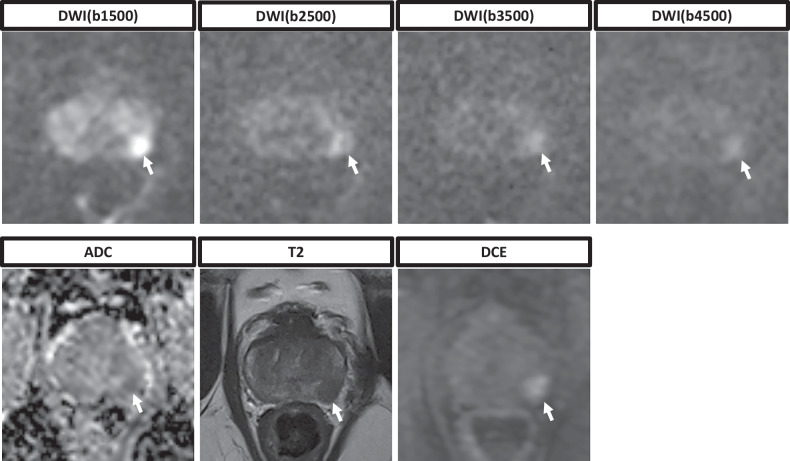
Representative images of a 61-year-old patient. The patient presented with an elevated prostate-specific antigen of 4.27 ng/mL. The whole multiparametric MRI protocol is shown, consisting of T2-weighted sequences, DWI sequences with calculation of the ADC map, and DCE sequences. The suspicious lesion in the posterolateral peripheral zone can be clearly delineated in the DWI(b1500), whereas both contrast and signal-to-noise ratio decrease in the DWI(b2500), DWI(b3500), and DWI(b4500). All readers gave a PI-RADS score of 4 for both readings that included either the DWI(b1500) or DWI(b2500). ADC, Apparent diffusion coefficient; DCE, Dynamic contrast enhanced; DWI, Diffusion-weighted imaging; MRI, Magnetic resonance imaging; PI-RADS, Prostate imaging reporting and data system
